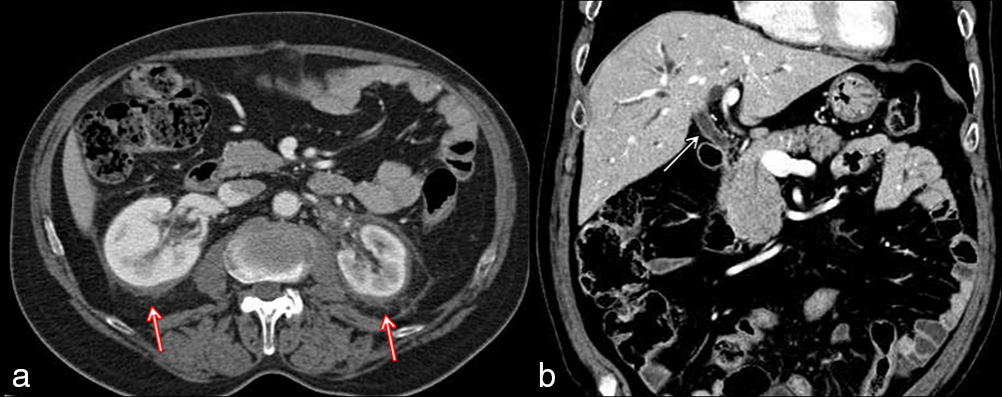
(a) Axial portal-phase CT scan performed after 3 months of medical therapy shows a marked reduction in size of the perirenal tissue on both sides (red arrows). (b) Coronal portal-phase CT image showing a similar reduction in size of the peribiliary tissue (white arrow); a residual ectasia of intrahepatic bile ducts was observed.
